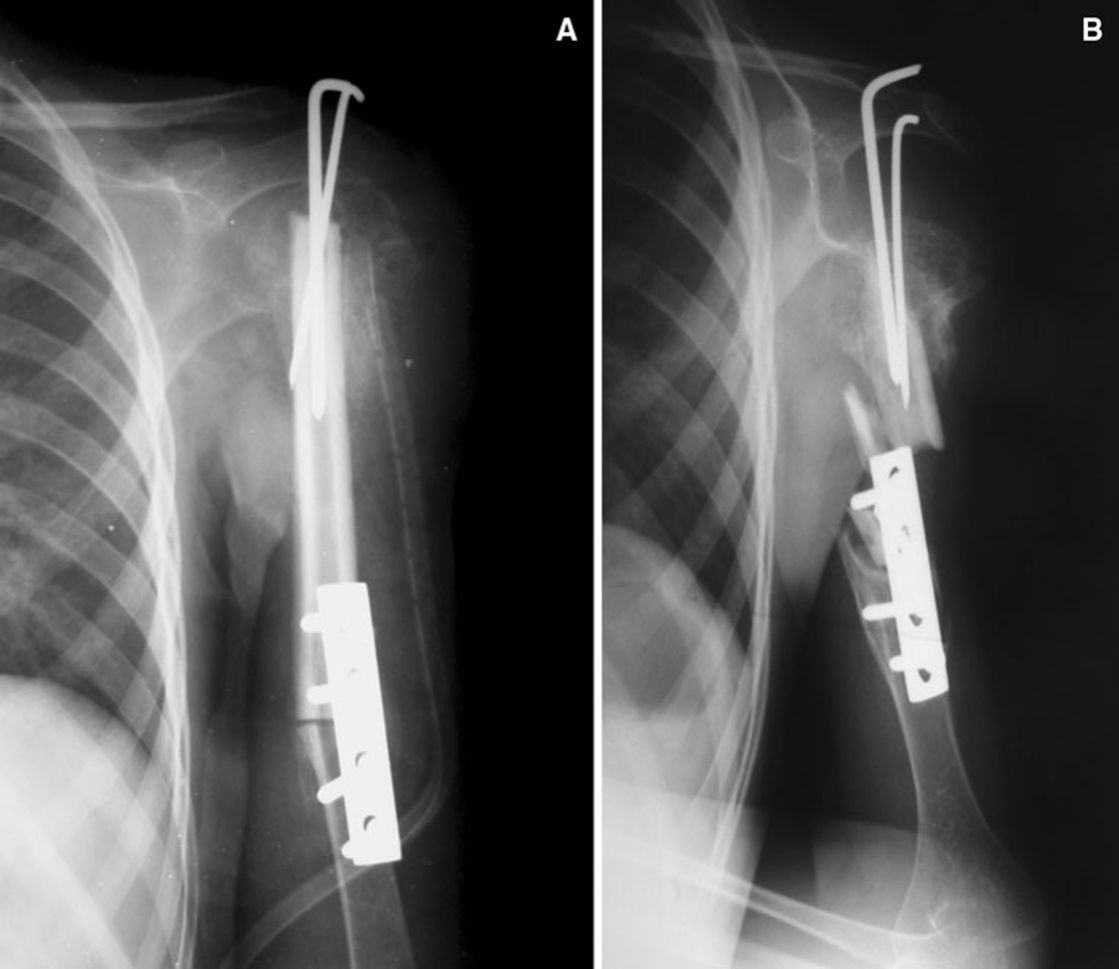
Plain radiograph after initial operation (a) indicates en bloc resection of ABC and reconstruction with cortical strut allograft. One year after the index operation, anteroposterior radiograph (b) shows failure of fixation with fracture of strut allograft and nonunion of the humerus
